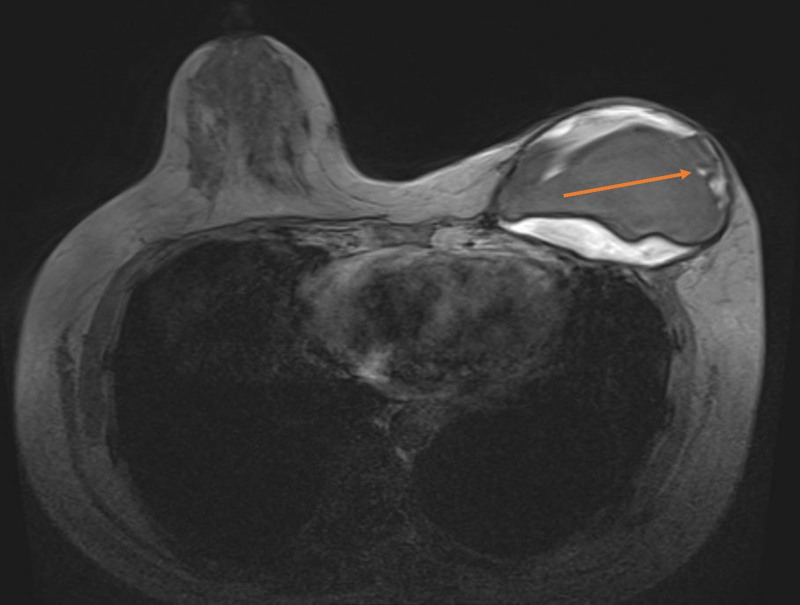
Breast MRI revealed fibrous capsule contracture surrounding the left mammary prosthesis, intracapsular effusion, suspicious silicone signal adjacent to the capsule, and rupture of the prosthesis shell, a suspicious tear drop sign can be observed (indicated by the red arrow). MRI = magnetic resonance imaging.
